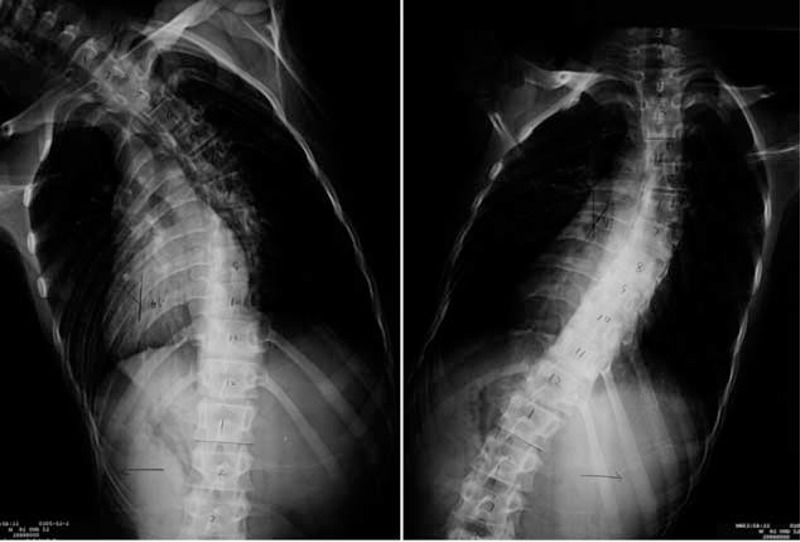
All length right and left side bending x-ray of spine on December 2009.
